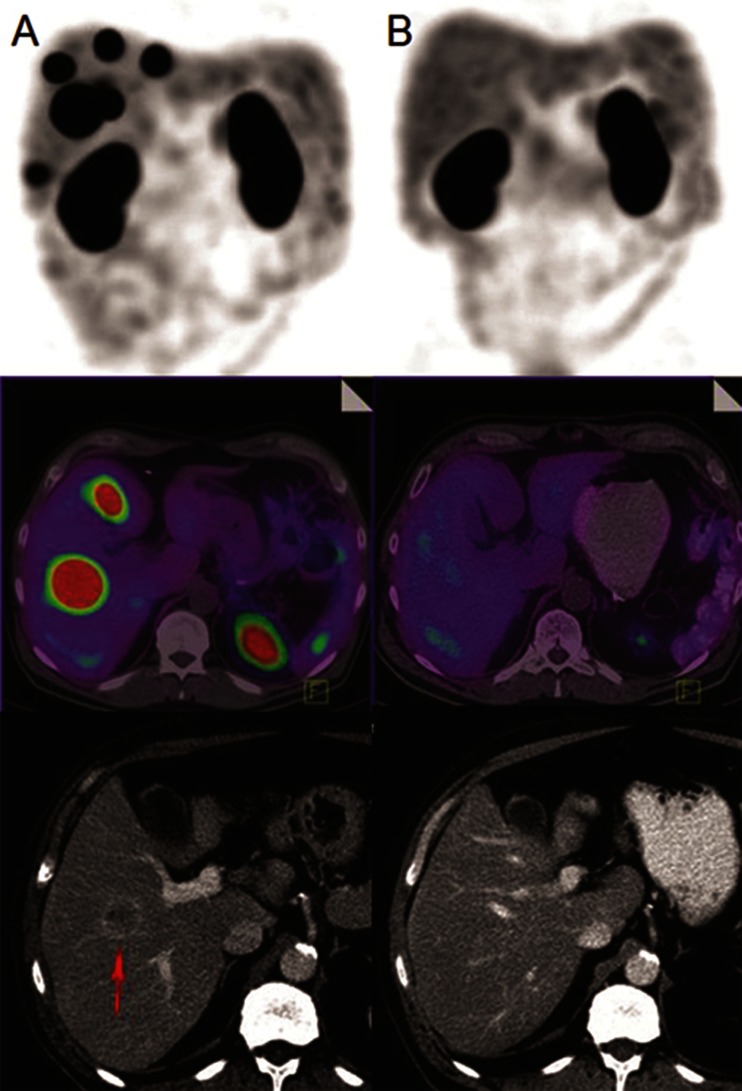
A 63-year-old patient with previously resected primary pancreatic NET referred for LuTate therapy due to progressive disease on CT in relation to multiple SSTR-positive liver metastases. *Top* Maximum intensity projection images. *Centre* Fused transaxial SPECT/CT images. *Bottom* Contrast-enhanced CT images. a After the first cycle of LuTate therapy at treatment baseline, the images show high SSTR-expressing liver metastases (*red arrow* most dominant lesion). b Complete scintigraphic and anatomical CT response to treatment approximately 3 years after induction LuTate therapy without any intervening treatments and despite only a partial early response. This patient remained disease-free at the time of this report
